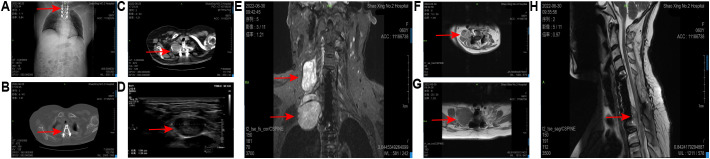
Imaging findings following the patient’s second recurrence and prior to initiating second-line therapy. (A-C) A chest CT scan on June 23, 2022; (A) Metallic shadow observed postoperatively on CT localization image; (B) Metallic shadow observed postoperatively on CT horizontal section image; (C) A soft tissue lesion located in the right cervical region, with involvement of the adjacent right first rib; (D) A lymph node located in the right supraclavicular region, with the largest dimension measuring approximately 19.4 × 12.5 mm, observed on an ultrasound image dated June 25, 2022; (E-H) An enhanced MRI of cervical spine conducted on June 30, 2022; (E) The lesions located on the right side of the cervical vertebrae indicated by the arrow above and on the right thoracic inlet indicated by the arrow blow exhibited long T2 signal intensities; (F) A lesion on the right side of the cervical vertebrae exhibited long T1 signal intensities; (G) A lesion on the right thoracic inlet exhibited long T1 signal intensities; (H) A lesion located at the posterior margin of the cervical vertebra, applying pressure on the cervical spinal cord.
